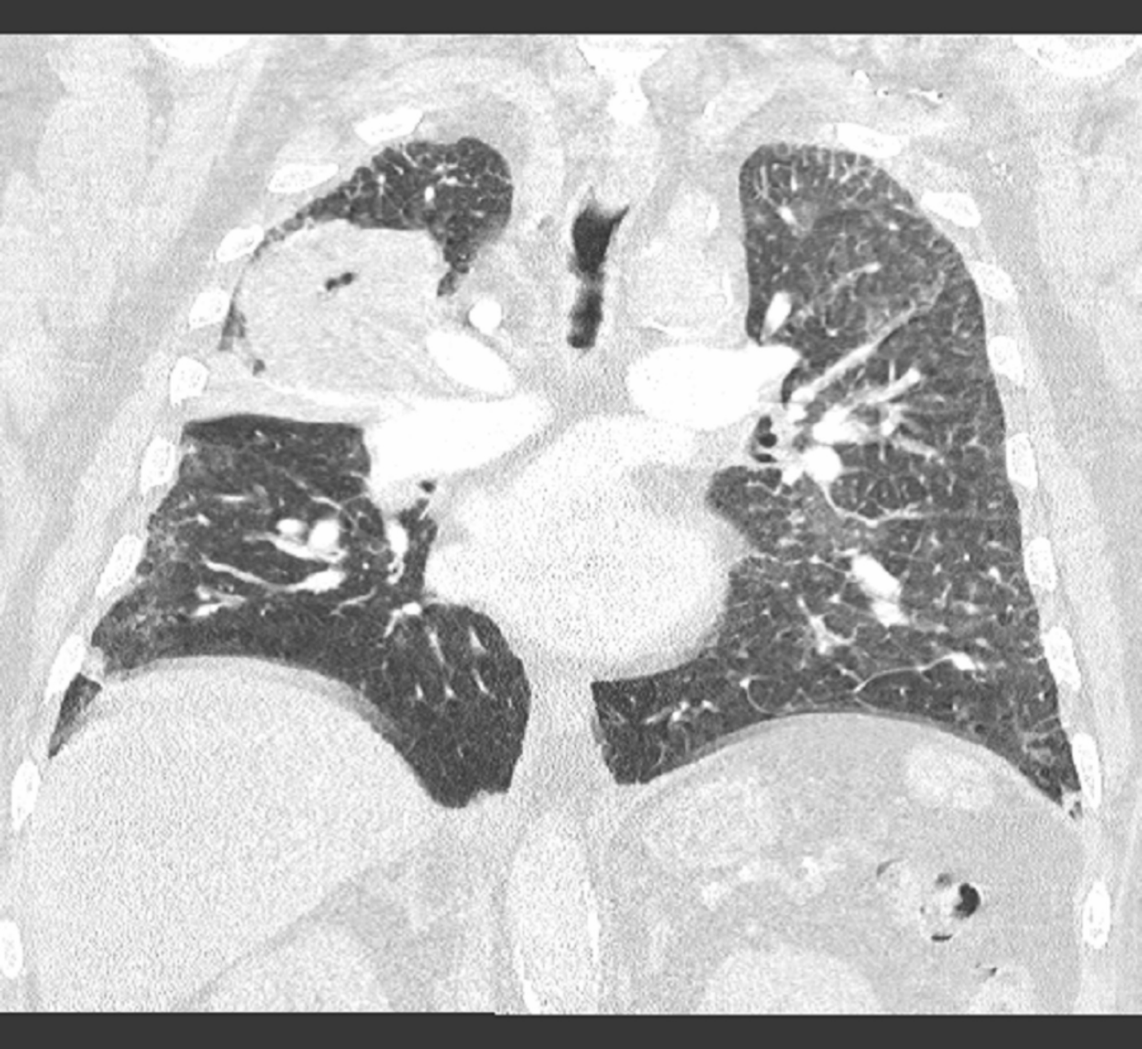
Coronal View of Computed Tomography Chest
